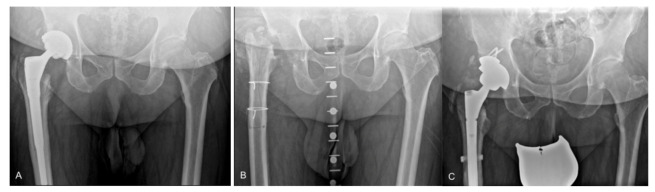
(A) Anteroposterior radiograph of a 68-year-old male patient with culture negative periprosthetic infection of the right hip showing a well-fixed cementless long stem 6 years after implantation. (B) Radiograph taken after first-stage total hip arthroplasty (THA) removal using an extended trochanteric osteotomy (ETO) with cerclage fixation without spacer insertion. (C) Postoperative radiograph showing the reimplantation after 7 weeks utilizing a highly porous metal shell with augment and modular fluted tapered stem. At the time of reimplantation, ETO was successfully healed and cerclages were exchanged.
